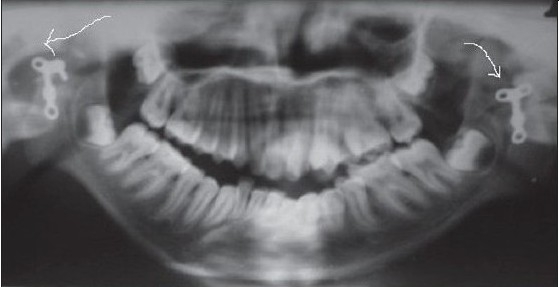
Postoperative orthopantomogram showing bilateral interposition of T-plates (arrow)
